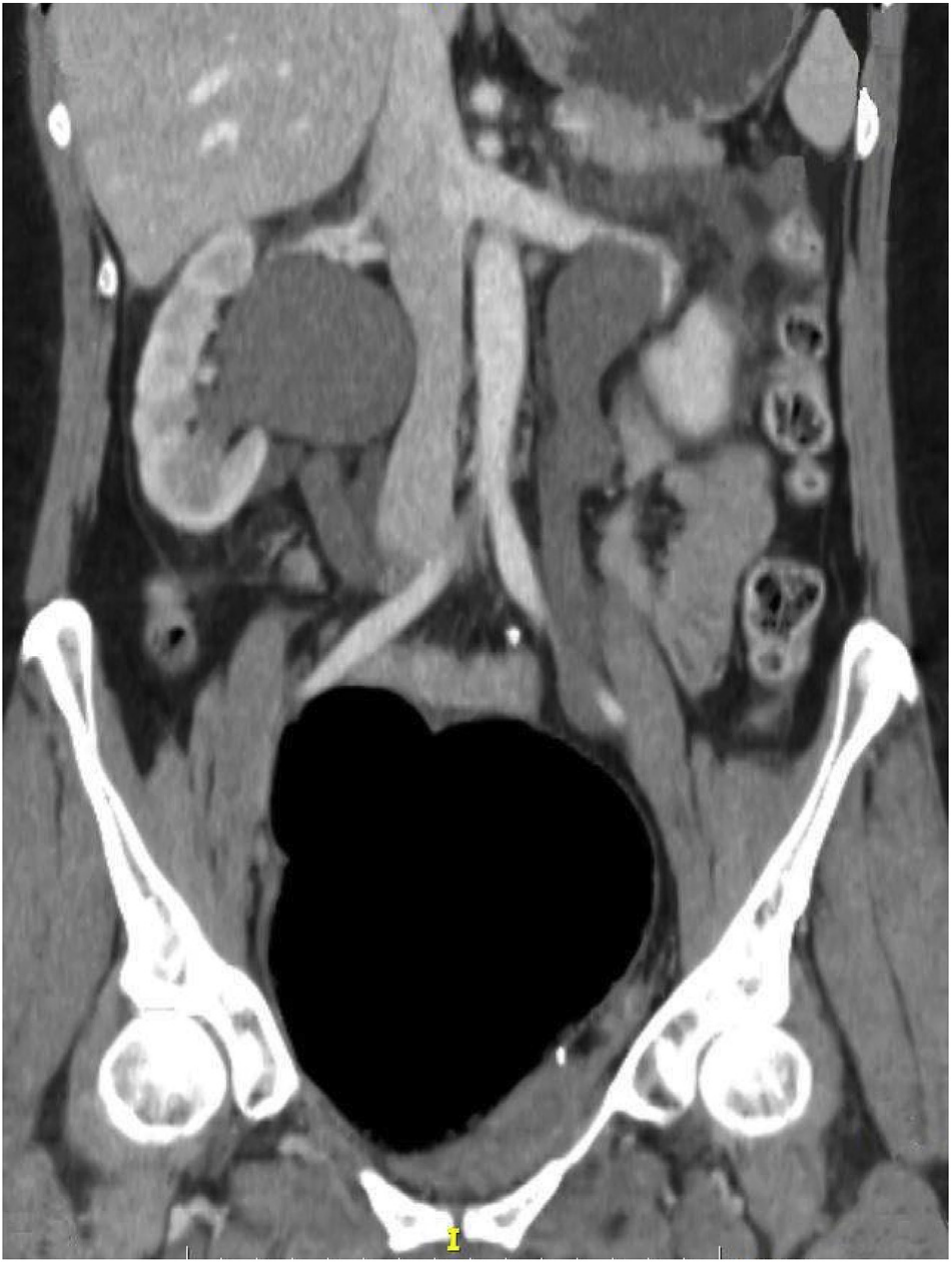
Axial CT image depicting obstructive uropathy due to gas-filled pelvic structure.
